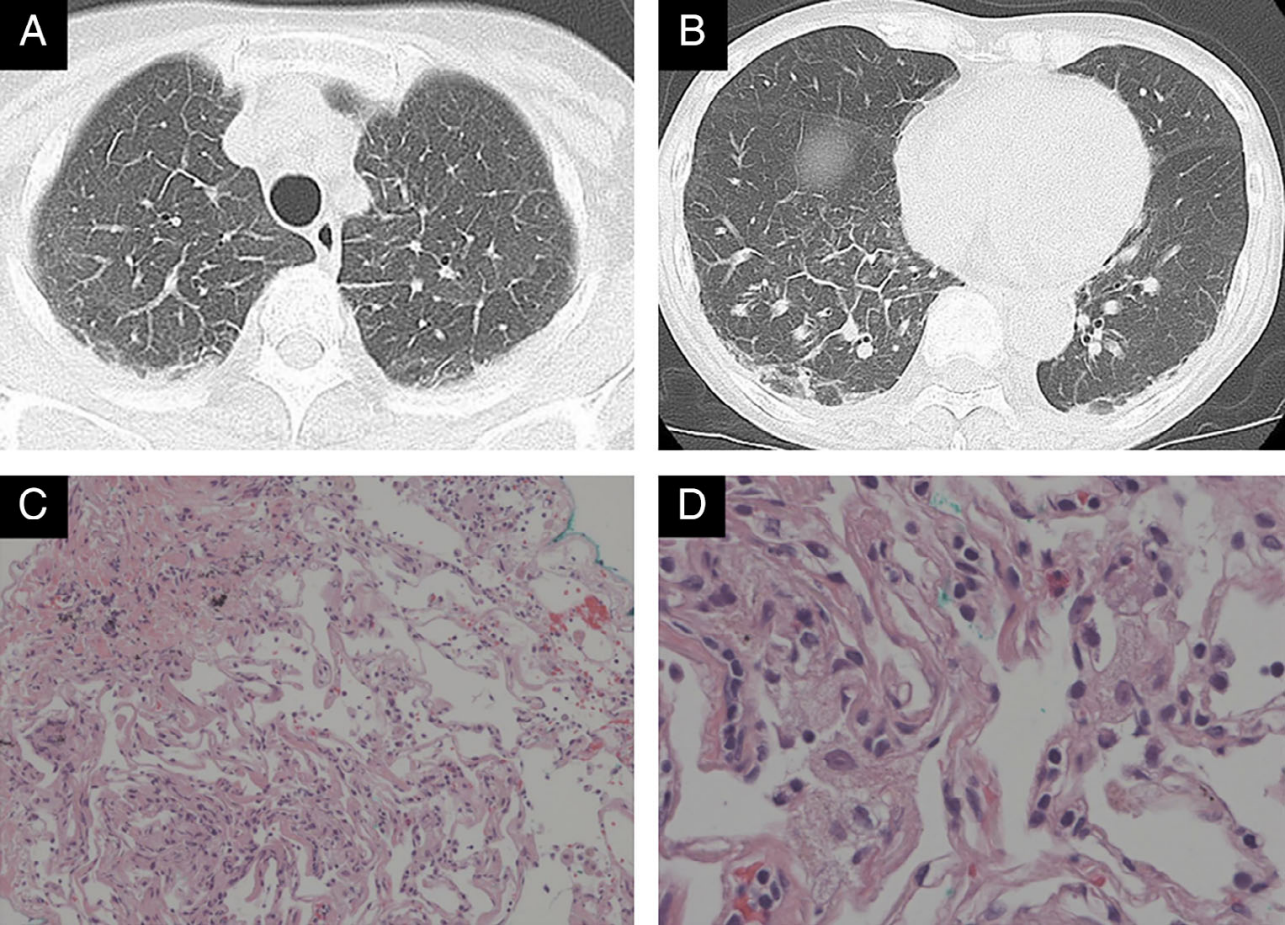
Chest computed tomography (CT) findings on admission. Chest CT demonstrated non‐segmental subpleural consolidation, ground‐glass opacities, forming reversed halo sign in some areas, and interlobular septal thickening, without pleural effusion (A, B). Subpleural consolidation and interlobular septal thickening were predominant in bilateral lower lobes (B) over upper lobes (A). Pathological findings of transbronchial lung biopsy were non‐specific with lymphocyte infiltration into interlobular septum (C, D), without caseous necrosis which is specific to tuberculosis. Magnification power was 100× and 400× in (C) and (D), respectively.
